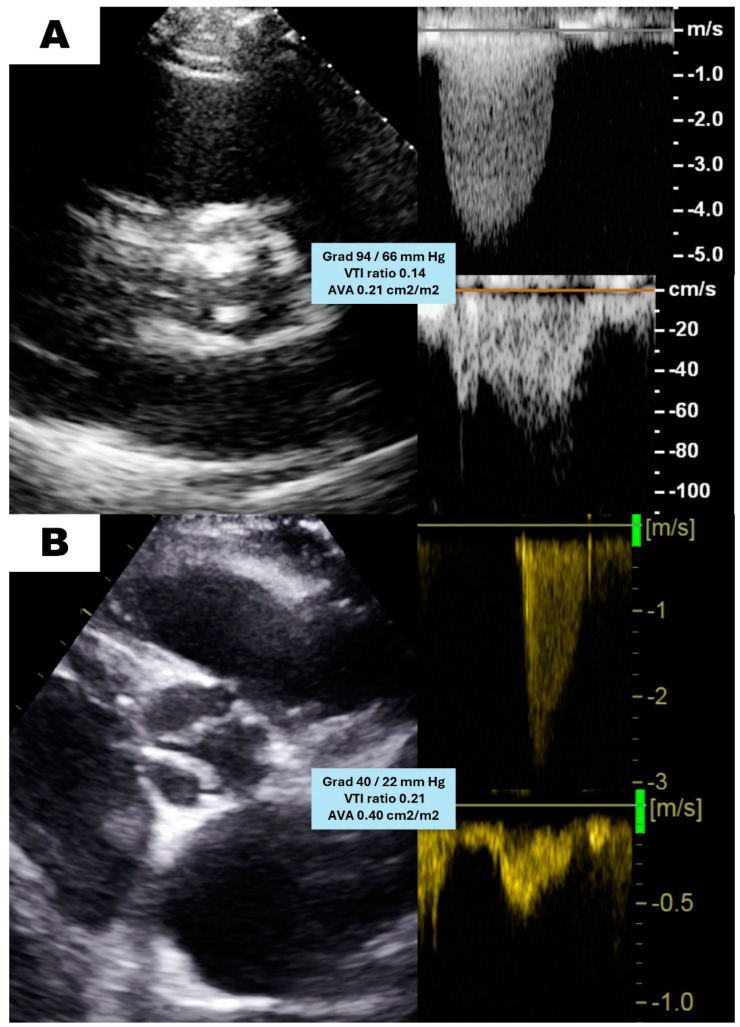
Echocardiographic features of the different patterns of aortic stenosis. Morphological and functional differences between high gradient (A) and paradoxical low-flow low-gradient (B) aortic stenosis. Please note that both forms result in a severely reduced aortic valve area regardless of the gradients.
